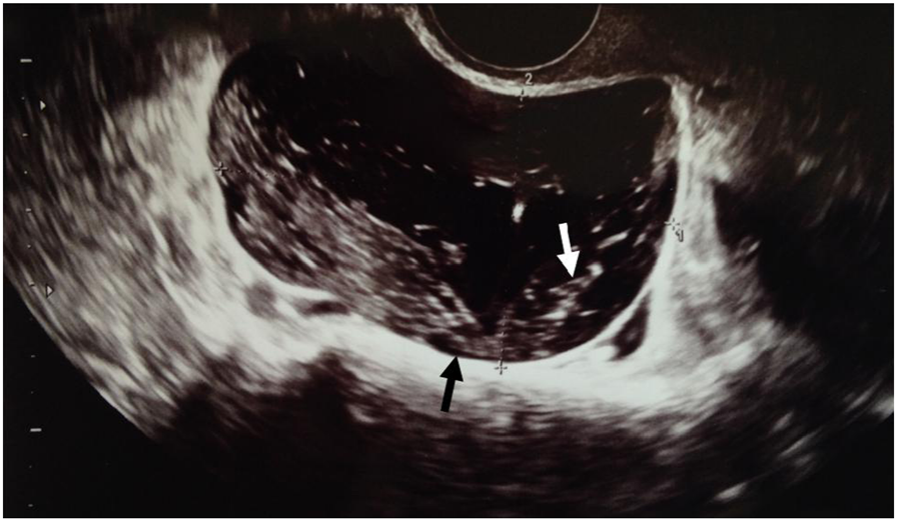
Transvaginal ultrasonography. The right adnexal region revealed a unilocular cyst 7 cm in maximal diameter with a thin wall (black arrow) and a mixed internal echogenicity (white arrow).
